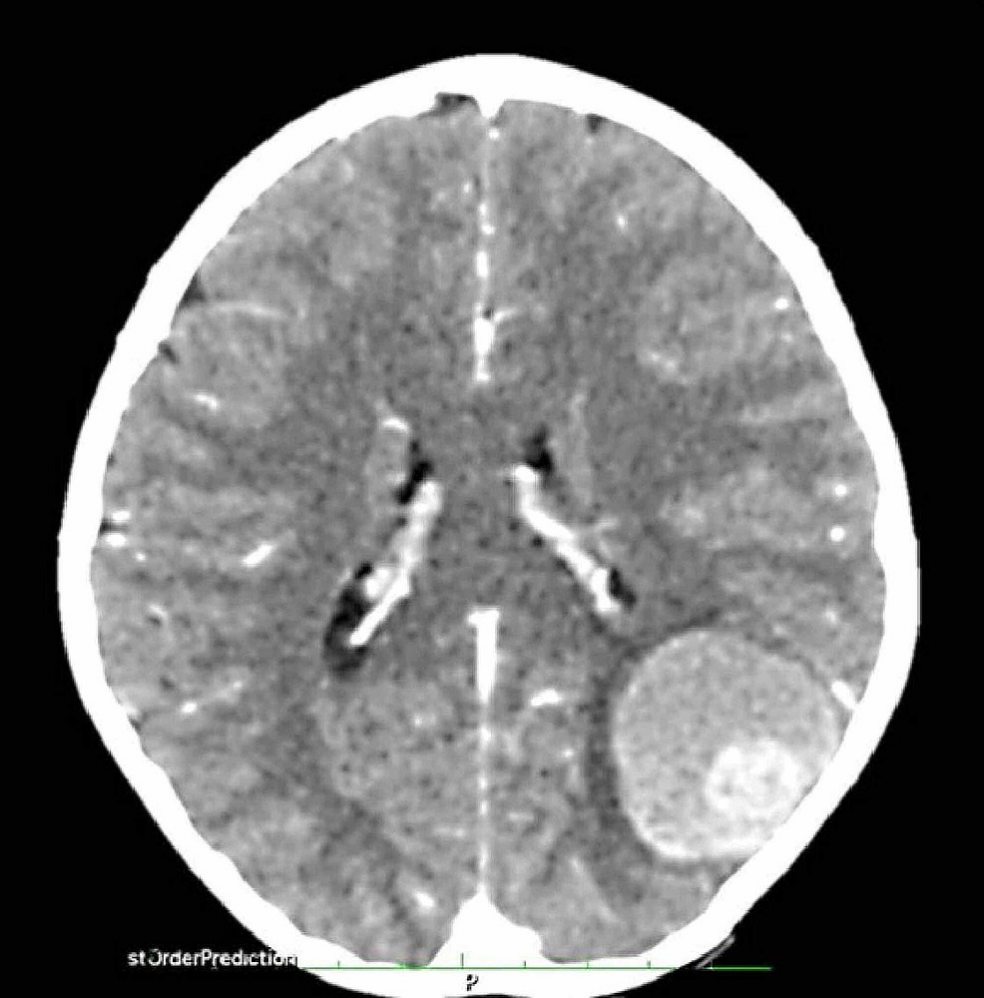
CT angiography image. Normal meningeal vessel enhancement can be seen. No obvious feeding artery was detected.
